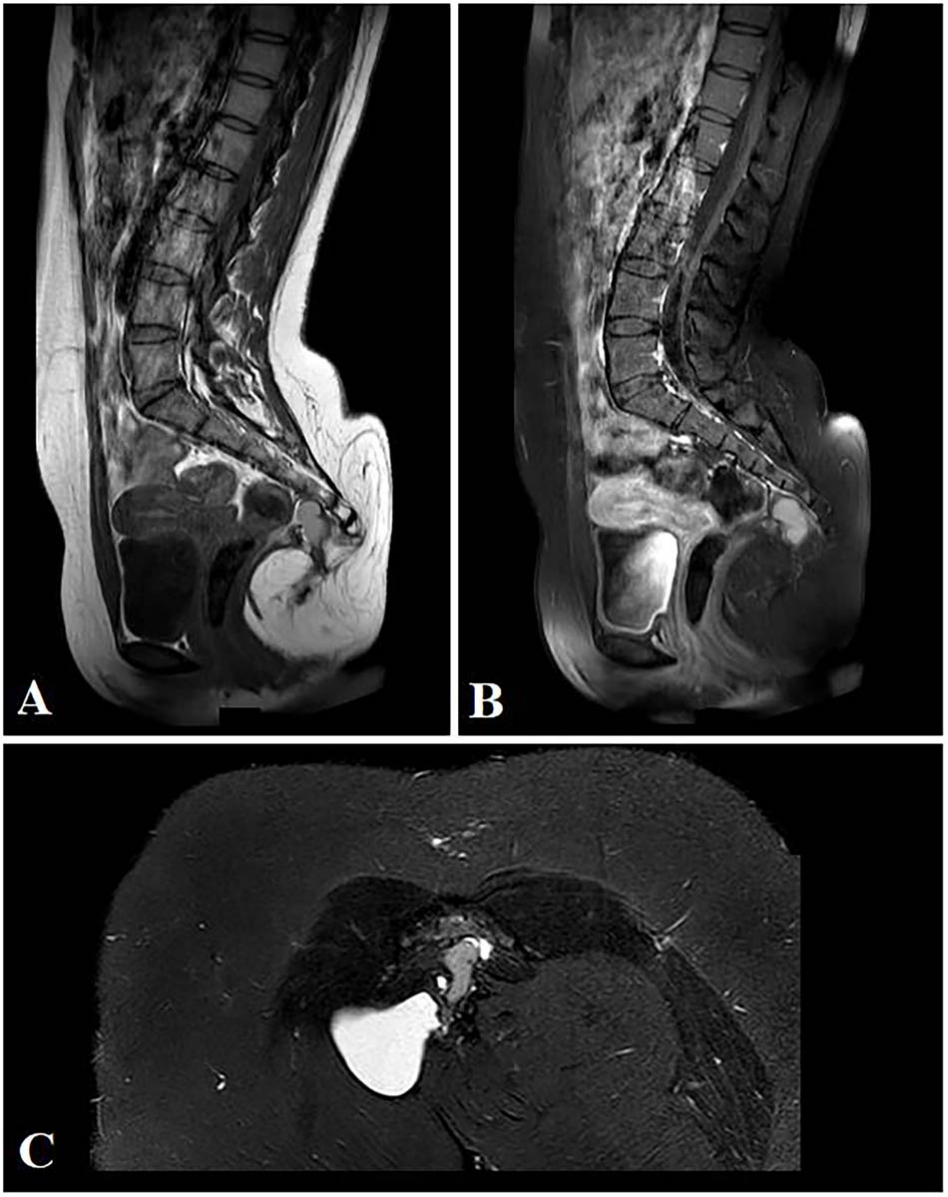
Selected sagittal MRI images (T1-sequence) without (A) and with contrast (B), both showing the extension of the mass with a visible pre-coccygeal component. The tumor is seen composed of mixed solid (predominantly fat) and cystic (fluid) components. The cystic component appears hyper-intense on the STIR sequence (C).
